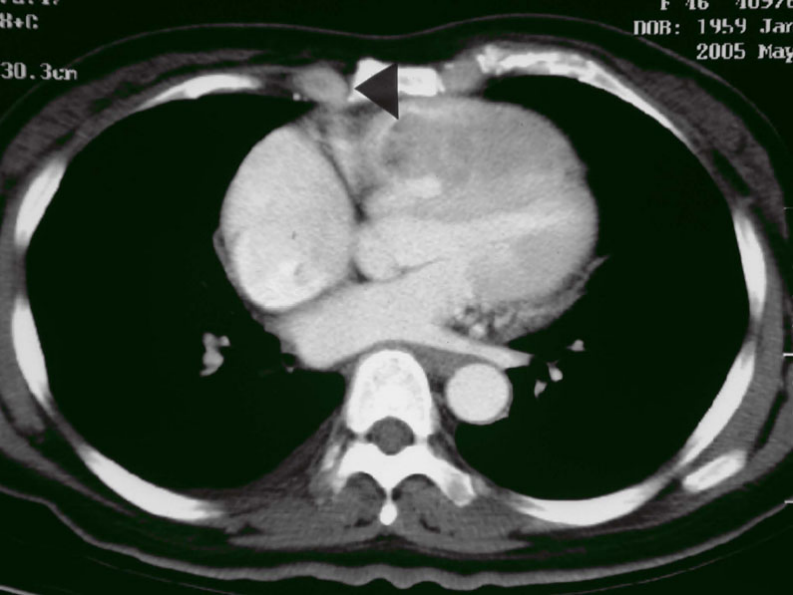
Computed tomography showing a mildly enhancing mass (arrowhead) surrounded by contrast medium. The mass was attached to the right ventricular wall, extending to the main pulmonary artery.
